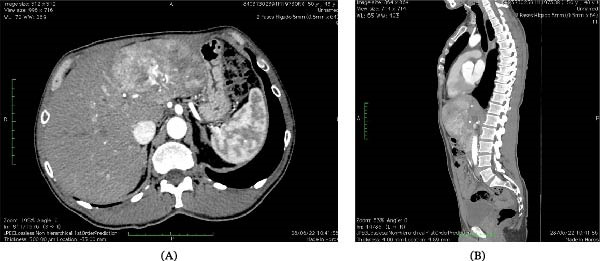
Contrast‐enhanced CT scan of the abdomen. (A) Sagittal section showing the longitudinal extension of the tumor from the T12 to L1 vertebral levels. The anterior displacement of the liver toward the abdominal wall can be observed. (B) Axial section showing the extension of the tumor involving liver segments II and IV. In both sections, the heterogeneous enhancement pattern of the mass can be appreciated.
